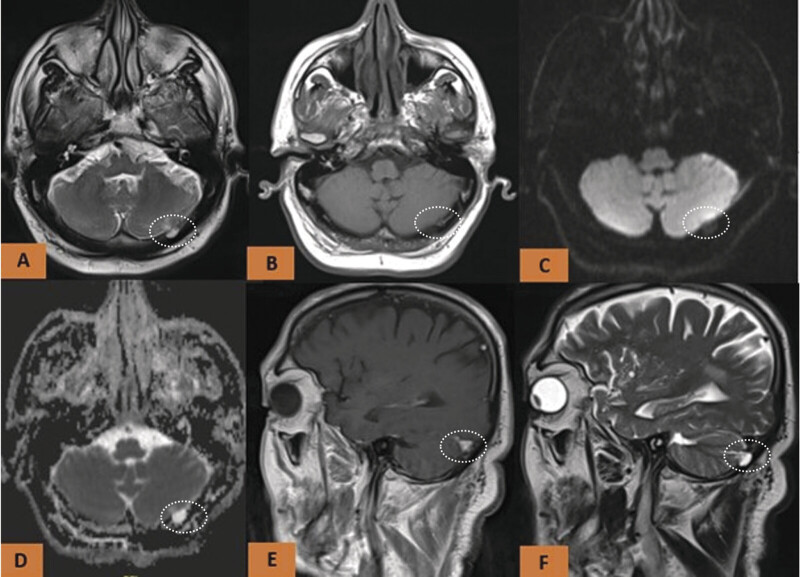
An intradiploic left occipital arachnoid cyst (a non-expansile cystic mass lesion measuring 10 × 6 × 7 mm) shows a hyperintense signal on the axial T2-weighted image and a hypointense signal on the axial T1-weighted MR image (white circles) (
